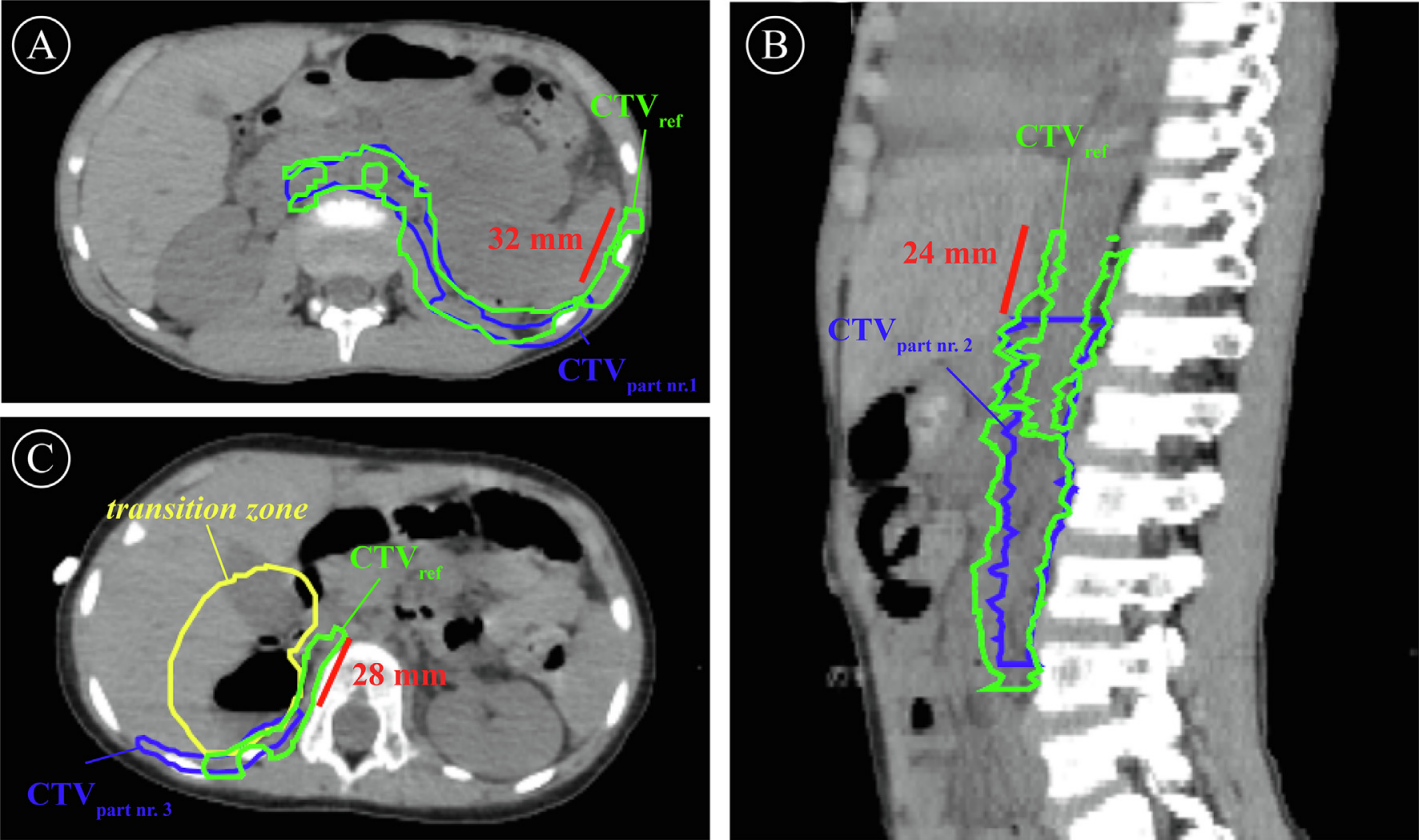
Three examples of an unacceptable variation by a participant observed during target volume review of case 5 and case 6. The postoperative CT’s show the CTV_ref_ (*green*) compared to the CTV of three different participants (*blue*) who were found to have an unacceptable variation measured with a point-to-point ruler (*red*). For case 5, participant number 1 underestimates the lateral margin of the CTV-T by 32 mm (4A, axial slice), and participant number 2 underestimates the cranial border of the CTV-N by 24 mm (4B, sagittal slice). For case 6, participant 3 underestimates the CTV-T at the medial side towards the transition zone (*yellow*) (4C, axial slice). (For interpretation of the references to colour in this figure legend, the reader is referred to the web version of this article.)
